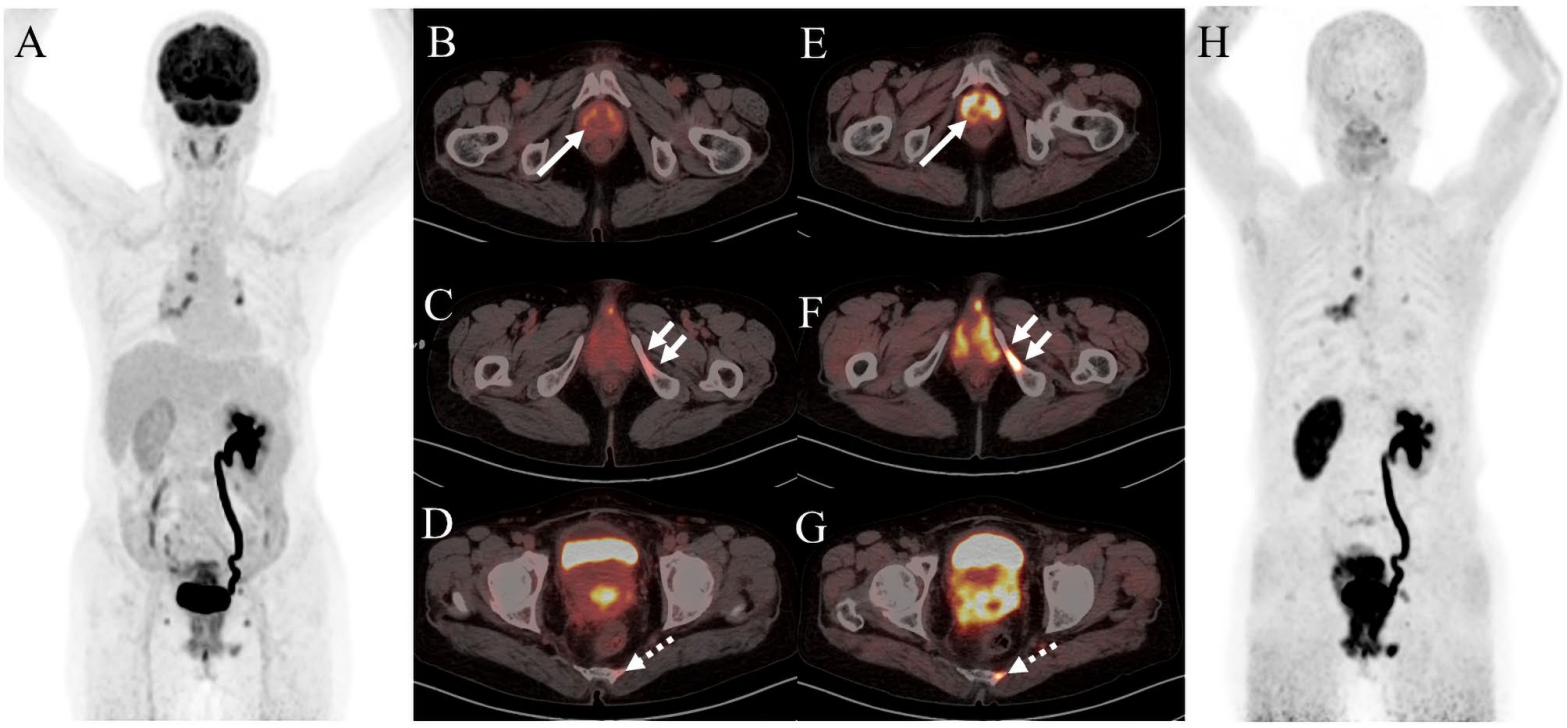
A 63-year-old woman was diagnosed with cervical squamous cell carcinoma in another hospital for more than 3 months and was referred to our hospital. Then ^18^F-FDG PET/CT and ^68^Ga-FAPI PET/CT were performed for initial assessment. The MIP image (A) and axial views of ^18^F-FDG PET/CT showed slightly increased uptake in cervix (B, solid arrow; SUV_max_, 8.6), left sacrum (C, solid arrow; SUV_max_, 2.4), and left ischium (D, dashed arrow; SUV_max_, 0.8). While ^68^Ga-FAPI PET/CT revealed significant increased FAPI uptake in corresponding cervix (E, solid arrow; SUV_max_, 12.5), left sacrum (F, solid arrow; SUV_max_, 11.1), and left ischium (G, dashed arrow; SUV_max_, 7.0). Subsequently, the patient received radiotherapy. It showed the left sacrum and left ischium lesion decreased in size after 3 months
